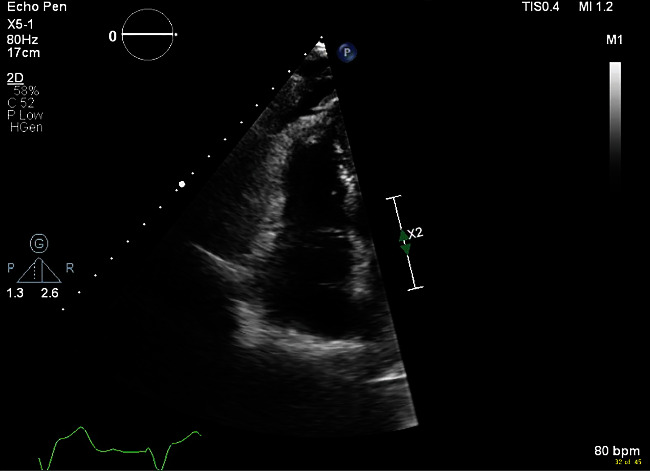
Transthoracic echocardiogram revealing pneumopericardium.
